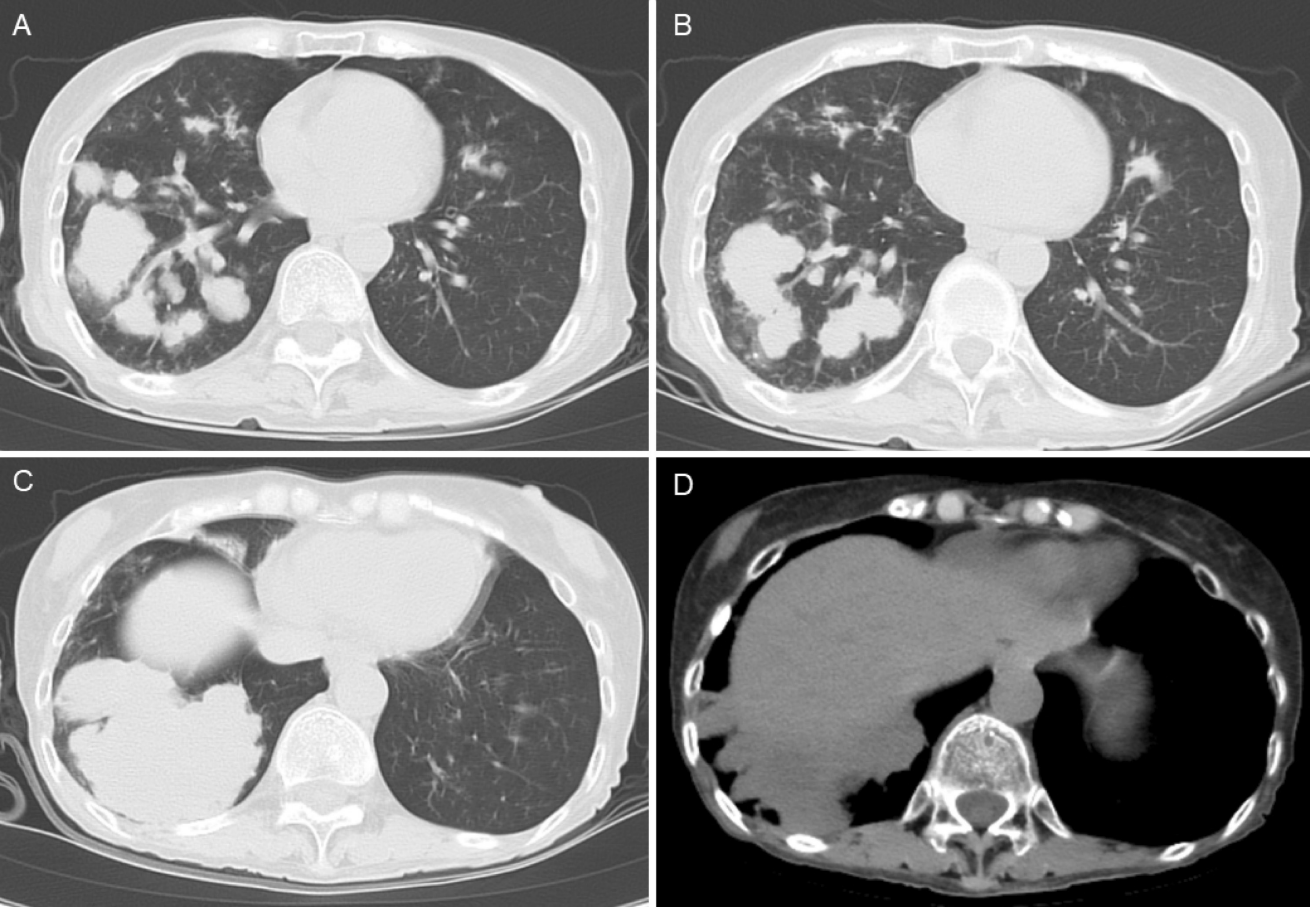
Chest computed tomography on admission demonstrating multiple irregular, mildly lobulated nodules and mass‐like lesions with relatively low internal attenuation in both lungs, predominantly in the right lower lobe, without cavitation or pleural effusion. (Panels A and B) show lobulated nodular lesions consistent with abscess formation. (Panel C) demonstrates a larger mass‐like abscess lesion. (Panel D) (mediastinal window) highlights the relatively low internal attenuation within the mass‐like abscess lesion.
